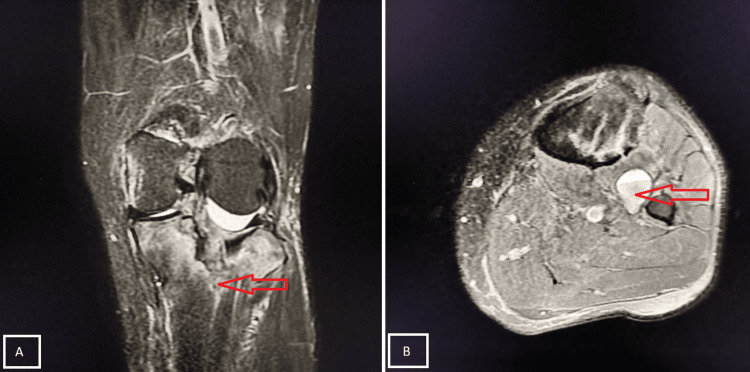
Schatzker Type II tibial plateau fracture Coronal STIR (A) MRI demonstrates a depressed fracture of the lateral tibial plateau (red arrow) with marrow edema and mild joint effusion consistent with Schatzker Type II fracture. Axial PD-FS (B) MRI shows the presence of an associated aneurysmal cyst (red arrow). PD-FS, proton density fat-suppressed; STIR, short tau inversion recovery
